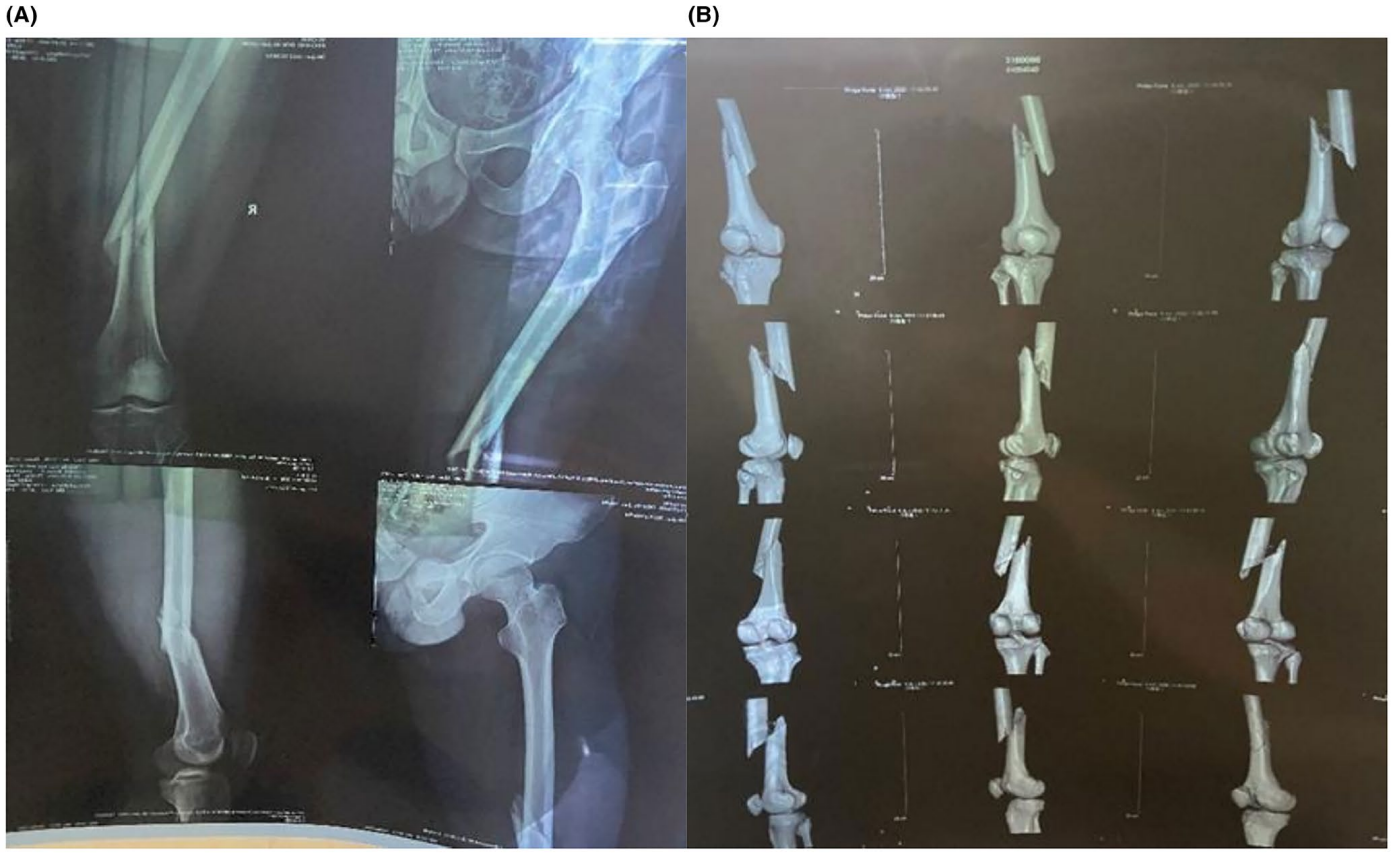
(A and B) X‐ray and CT imaging revealed a misaligned mid‐femoral fracture.
